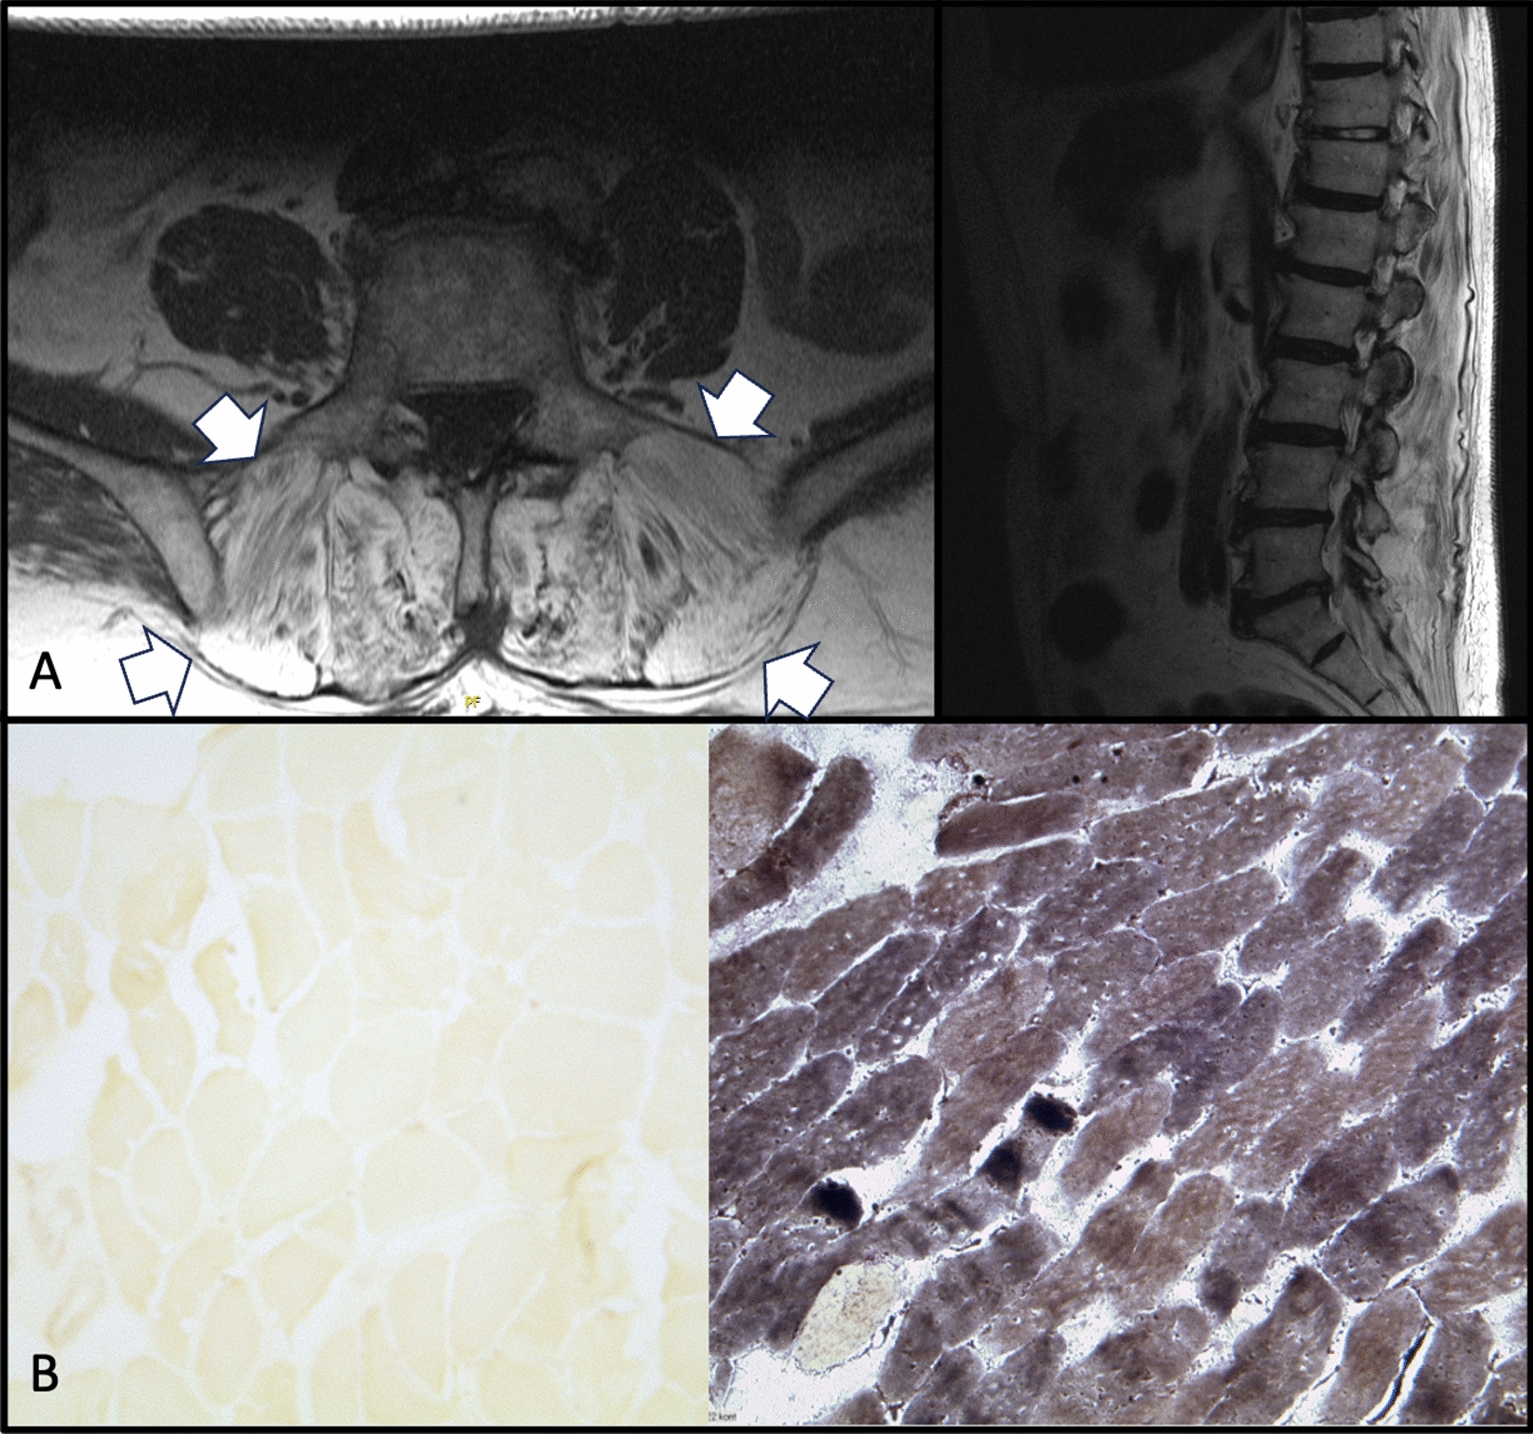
Magnetic resonance imaging scans demonstrate severe fatty degeneration of the autochthonous back muscles (white arrows) (T2w TSE transversal and sagittal. Siemens Symphony 1,5 T MRT) A Histochemical straining demonstrated the absence of myophosphorylase reaction in muscle fibres of the biopsy (left). Control sample with a normal myophosphorylase reaction in muscle fibres (right) (Left semitendinosus muscle, 10 × zoom, Histochemical enzyme reaction) (B). Written consent of the patient for publication of this photo was obtained
